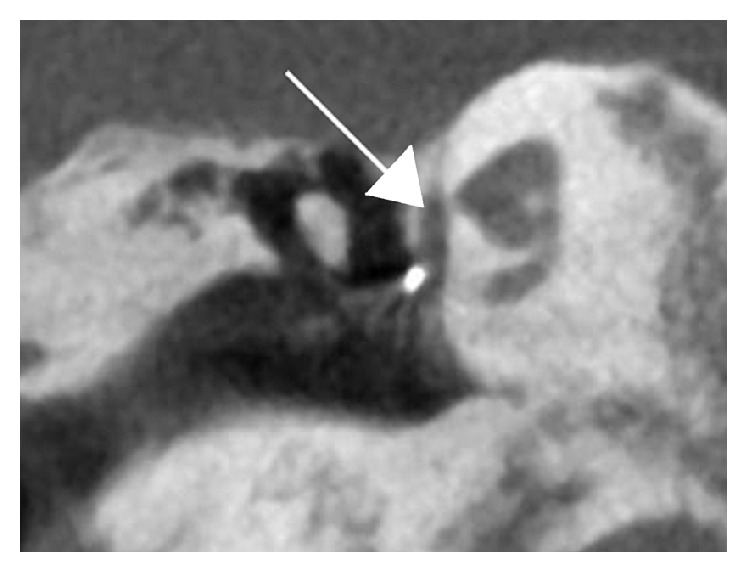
Coronal noncontrast computed tomography of right temporal bone reveals the end of a previously placed stapes prosthesis (metallic object) abutting the second genu of the facial nerve (arrow), which is descending anterior to the promontory as evidenced by its location adjacent to the turns of the cochlea.
